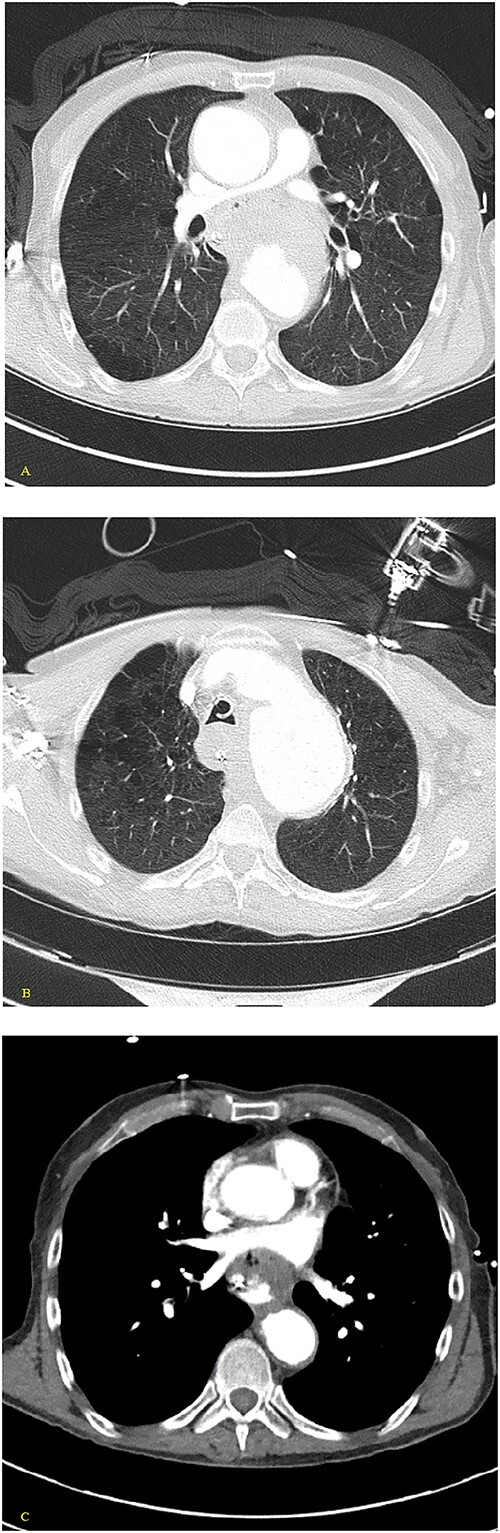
(A, B) Axial nonenhanced CT chest with contrast and (C) axial enhanced CT chest with contrast reveal an extensive clot around the aorta and around the esophagus with some mass effect on the pulmonary arteries and the LA. Clot fills the aorta.
